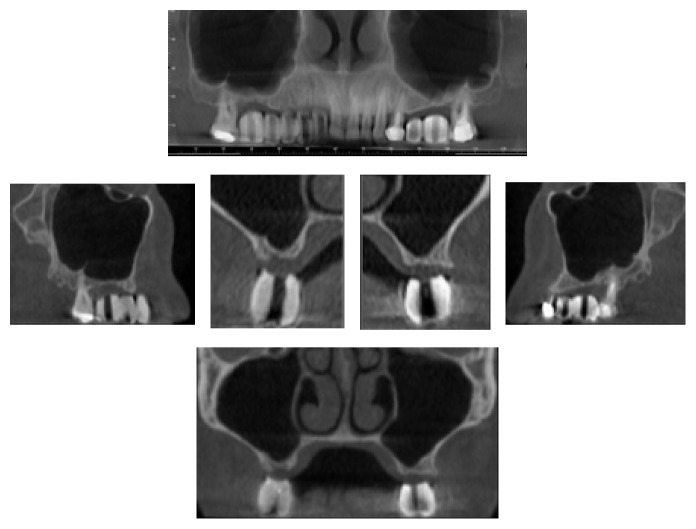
Baseline. The CBCT images show the posterior upper jawbone defect.
